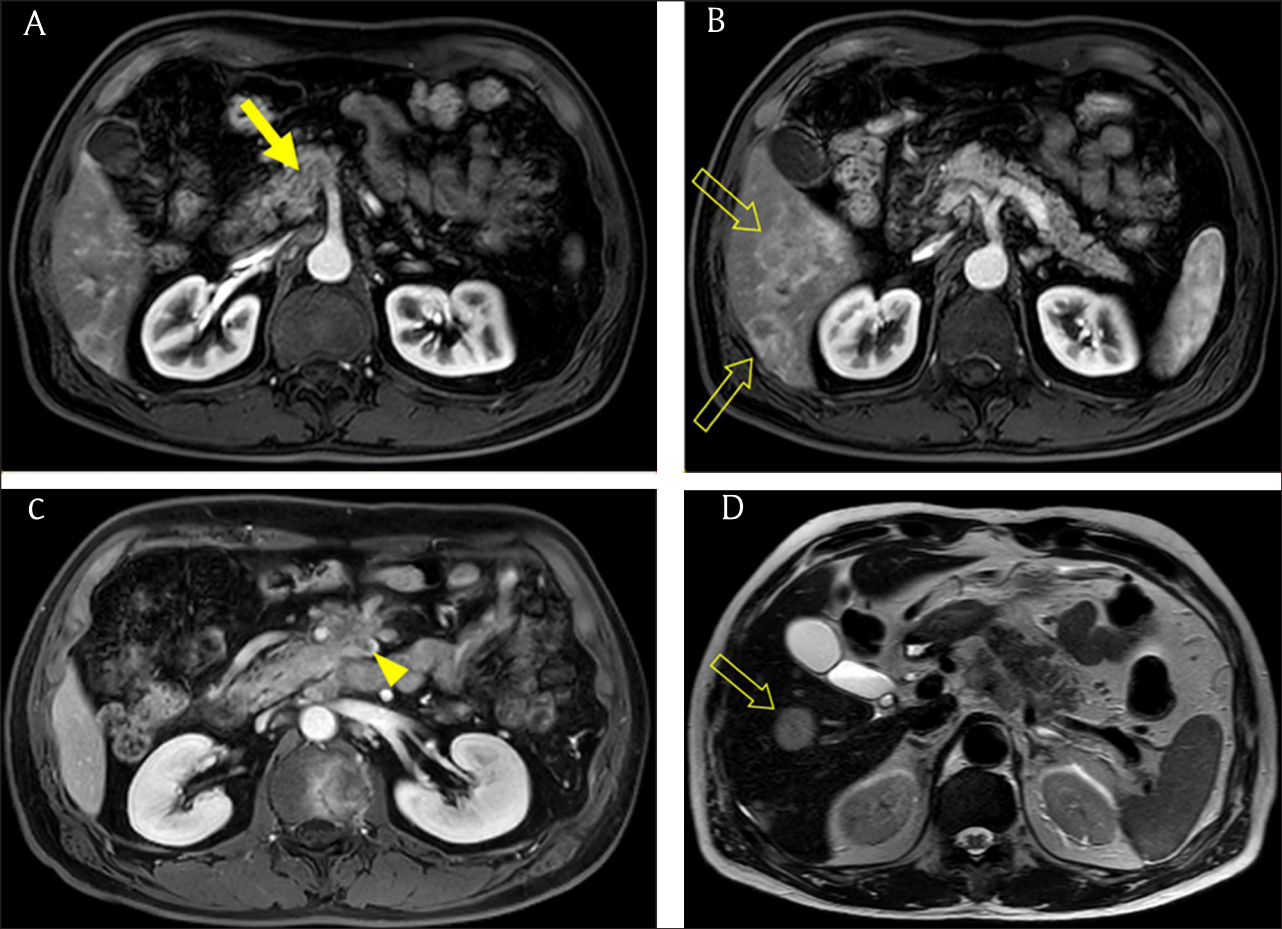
A 60-year-old man with a high-grade neuroendocrine tumor. (A, B) Axial gadoxetic acid-enhanced arterial phase MR images show an irregular mass (arrow) in the uncinate process of the pancreas. There are hypervascular masses (open arrows) in the right hepatic lobe. (C) Axial portal venous phase MR image shows tumor thrombosis (arrowhead) in a branch of the superior mesenteric vein. (D) Axial T2-weighted image shows a hyperintense mass (open arrow) in the right hepatic lobe. There is no dilatation of the pancreatic duct.
